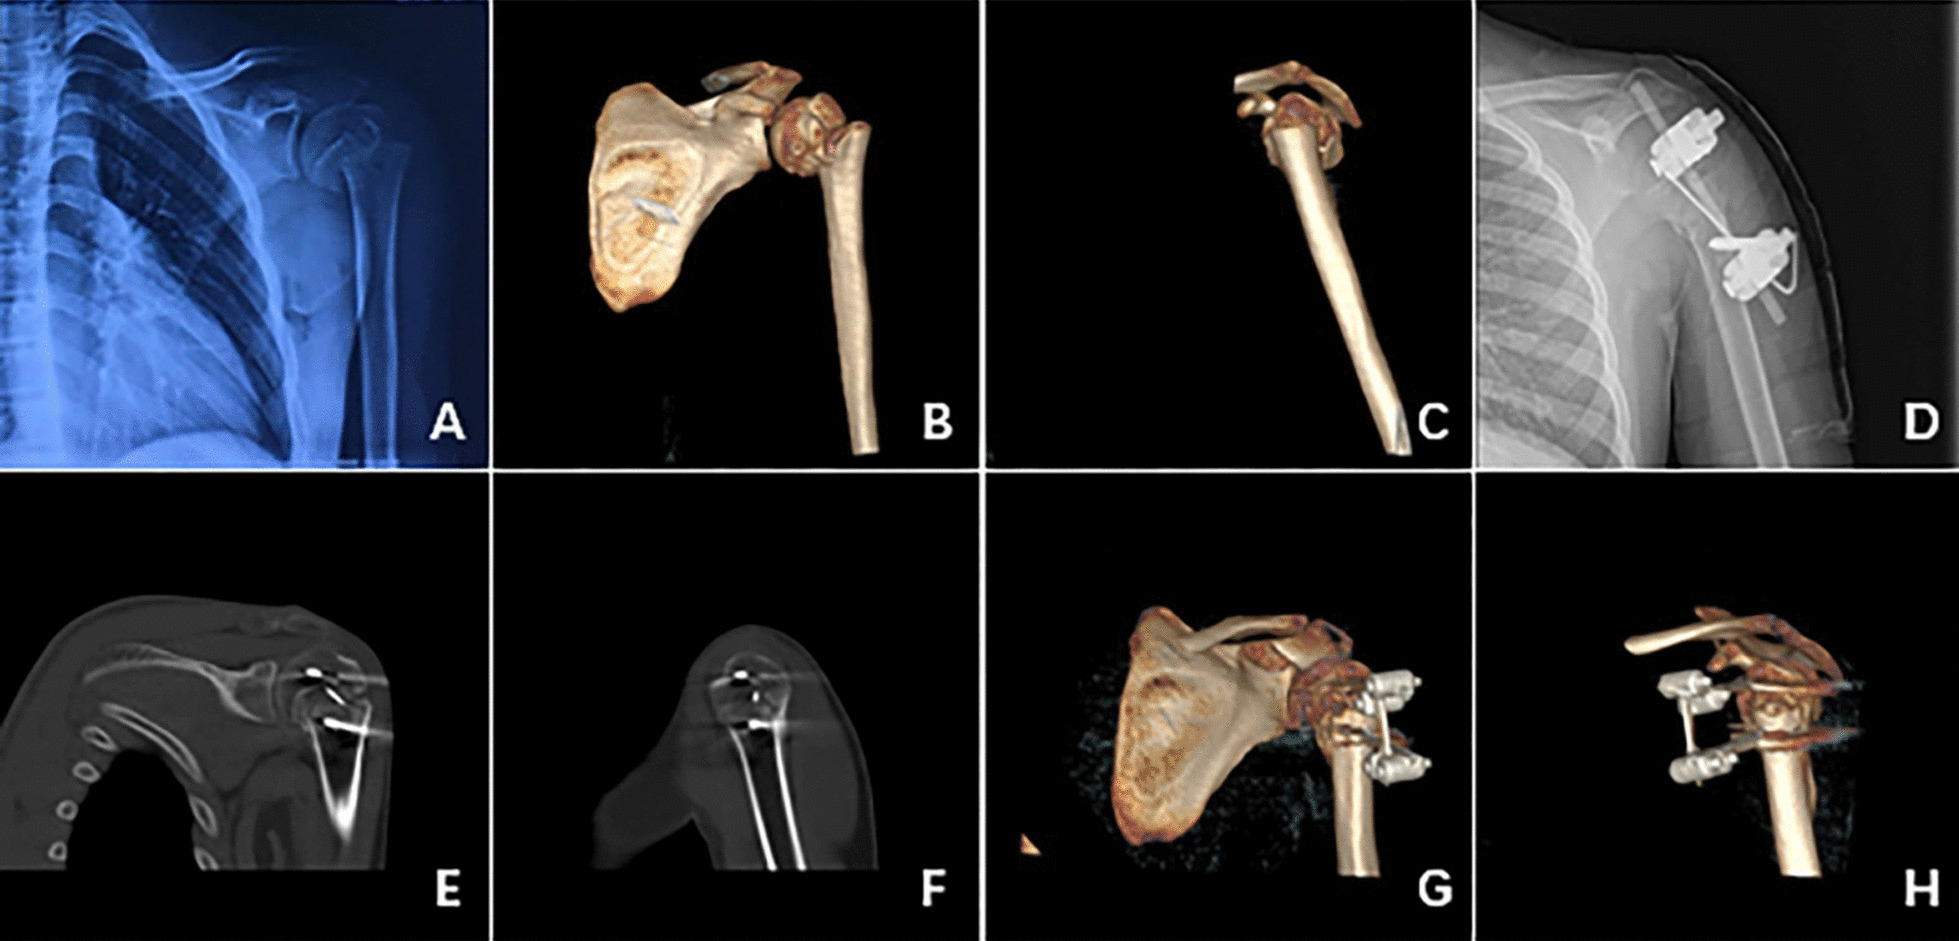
A Preoperative X-ray of a 15-year-old male patient with Salter–Harris II displaced proximal humeral fracture. B and C Preoperative 3D-CT of proximal humerus fracture. D Postoperative X-ray of proximal humerus fracture with the double-Schanz screw external fixator combined with anti-rotating Kirschner wire. E and F Postoperative 2D-CT of proximal humeral fracture (6 weeks after surgery). G and H Postoperative 3D-CT of proximal humerus fracture (6 weeks after surgery)
